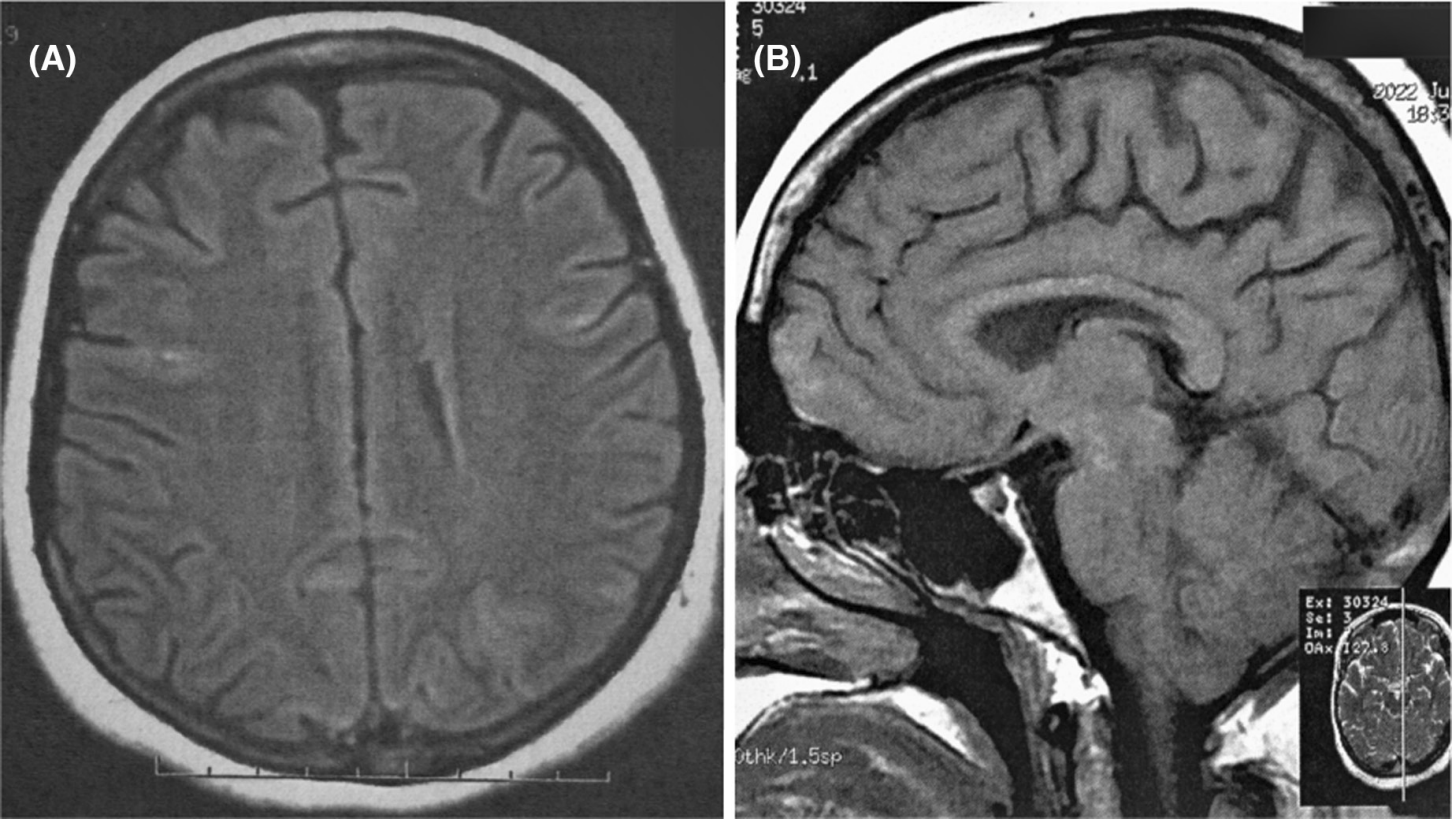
Axial FLAIR sequence reveals bilateral cortical hyperintensity (A), the sagittal T1‐weighted sequence shows no signal abnormality (B).
